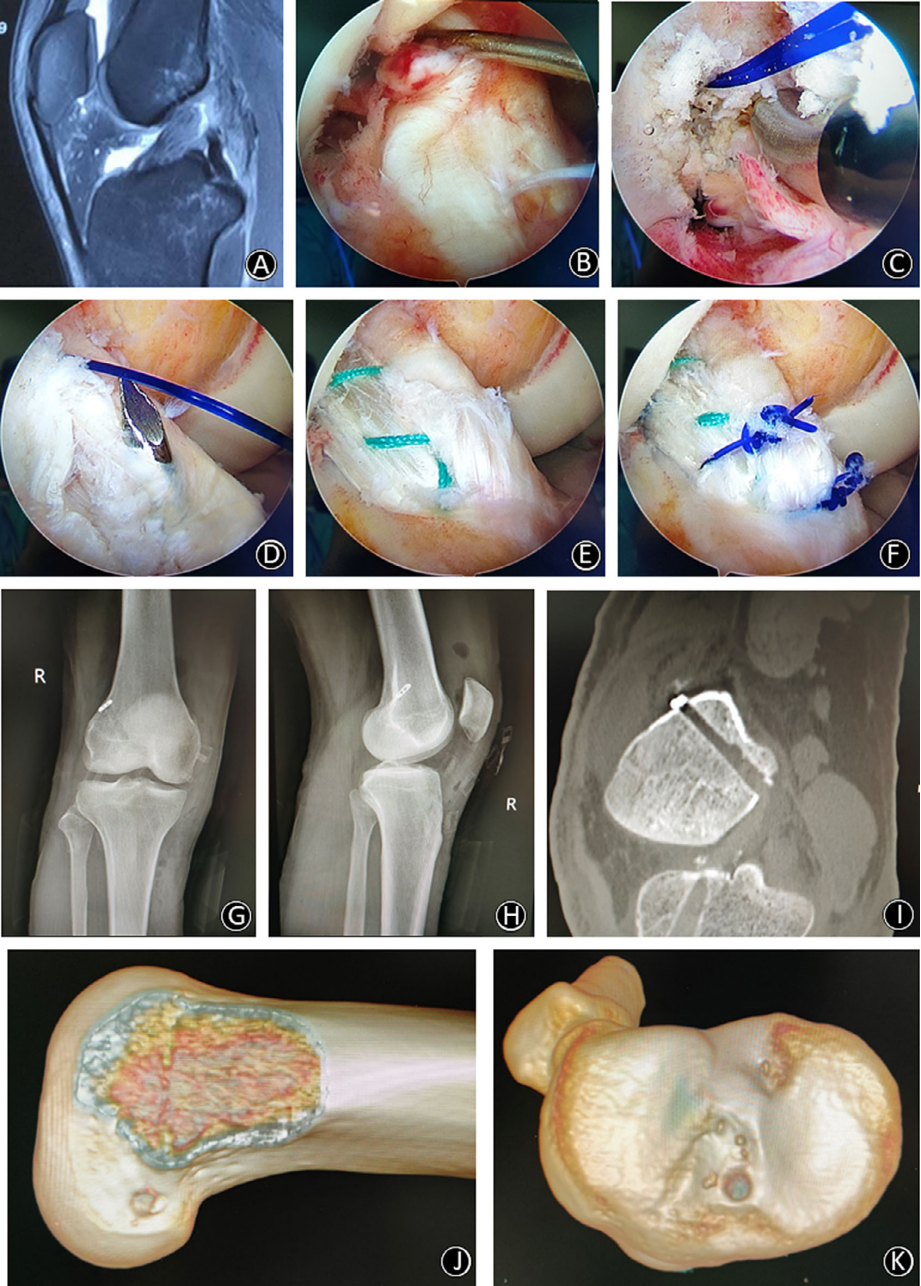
(A) MRI of the right knee showed rupture of the femoral end of ACL. (B) A routine arthroscopic examination was performed using a probe with a 30° oblique arthroscope in the anterolateral (AL) portal and another probe in the anteromedial (AM) portal. ACL avulsion from femoral insertion was observed. (C) Position of the I.D.E.A.L. femoral tunnel (arthroscopic view). A guide PDS was placed through the femoral bone tunnel. (D) The tibial tunnel (the position of the Kirschner wire) was located at the center of ACL remnant. (E) The position of ACL graft in the joint after fixation through the tibial and femoral tunnels. (F) The ACL graft and ACL remnant were sutured together with PDS suture. (G,H) Postoperative anteroposterior and lateral plain radiographs showed the correct position of the titanium plate. (I) Volumetrically rendered image from sagittal computed tomography taken after primary ACLR showed that the tunnels were located at the anatomic position. (J) 3D CT scan reconstruction with a femoral tunnel position. (K) 3D CT scan reconstruction with a tibial tunnel position.
